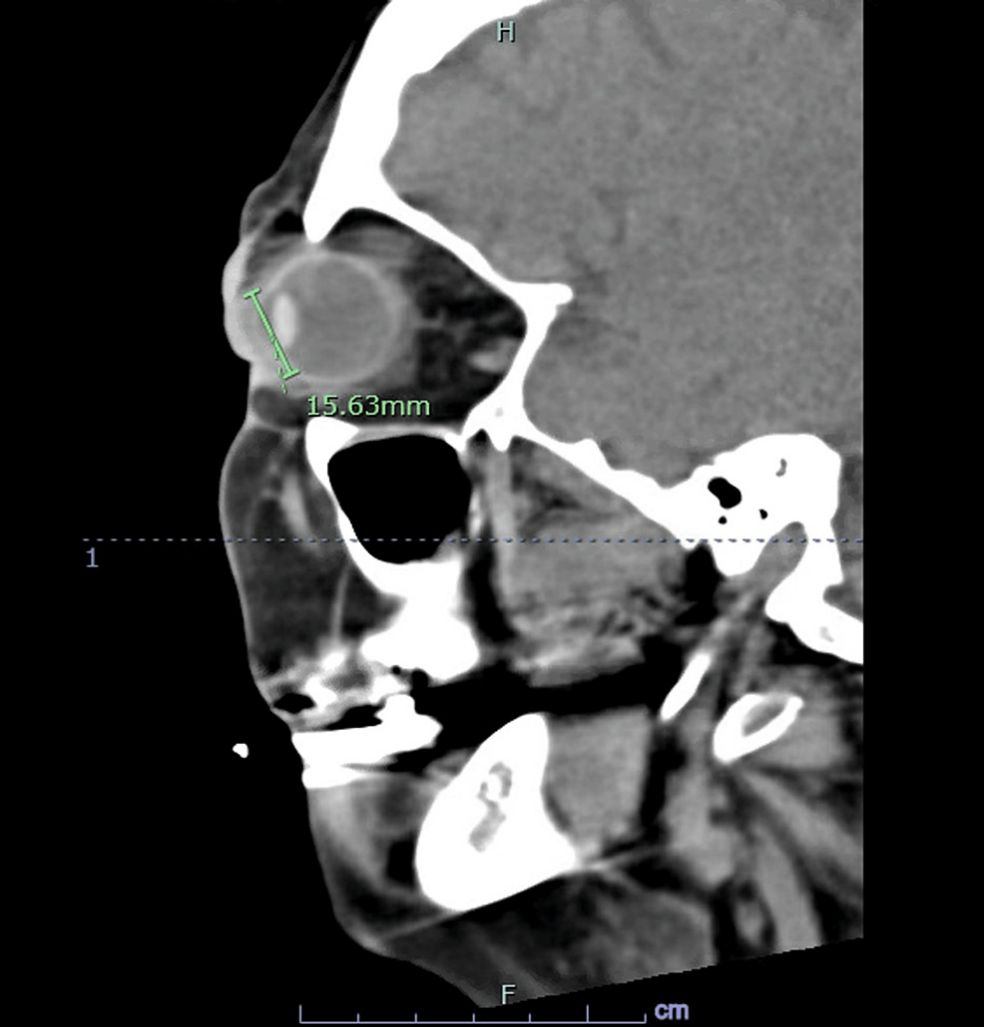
CT of the orbits shows stranding of retrobulbar intraconal fat and extraconal fat compatible with post-septal cellulitis and increased enhancement of the right optic nerve sheath complex and extraocular musculature, resulting in exophthalmos.
